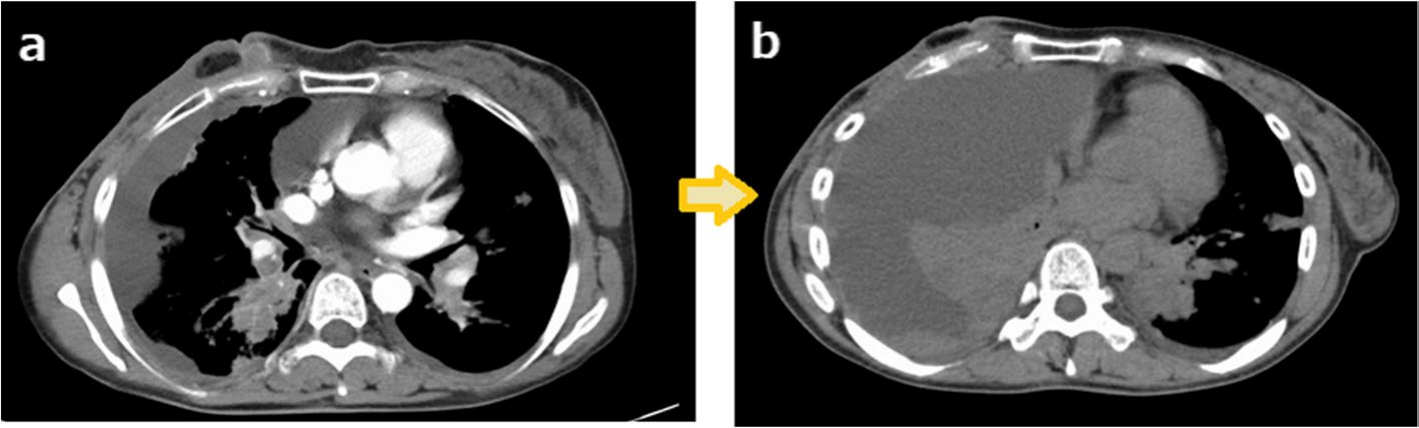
Contrast-enhanced CT performed at the time of recurrence demonstrates pleural effusion and dissemination (a). After one course of paclitaxel and bevacizumab treatment, pleural effusion was exacerbated and tumors in the lung were increased (b)
